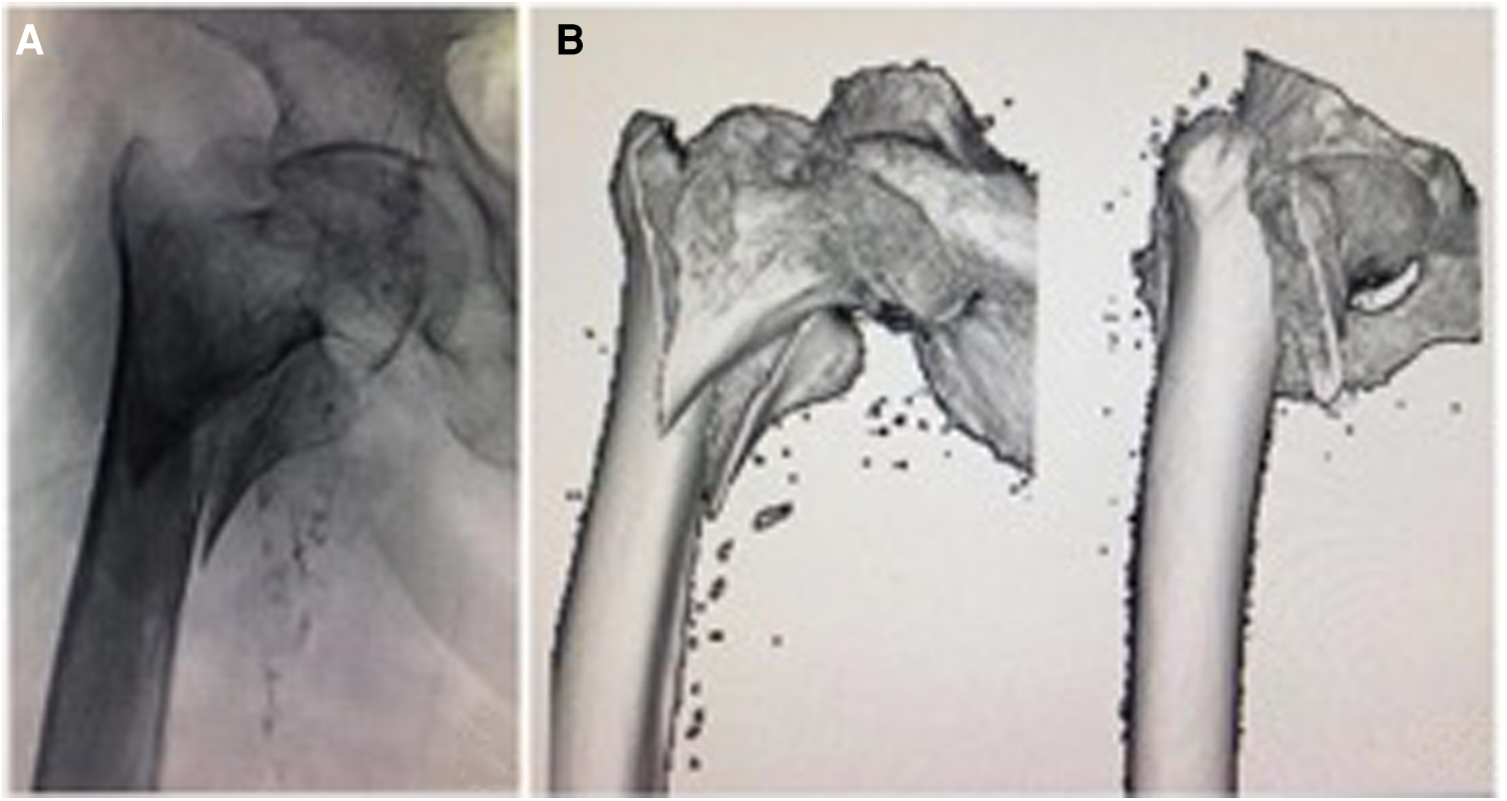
Intertrochanteric fracture in another patient, a 97-year-old woman, in which the medial arch was involved and displaced anteriorly. Preoperative x-ray (A) and CT scan (B) show that the intertrochanteric fracture and the medial arch fragment were displaced anteriorly.
